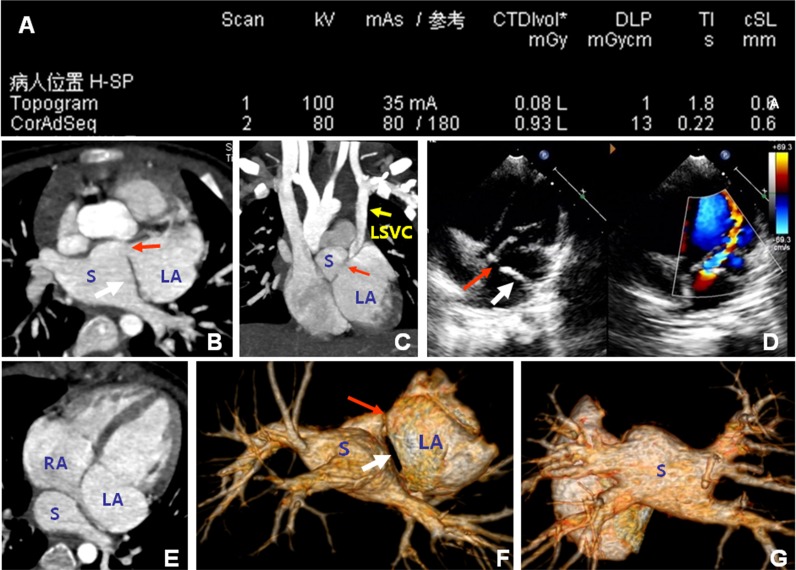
Representative multi-slice spiral computed tomography images from a female patient aged 2 years. A. Scanning parameters. CTDIvol, volume computed tomography dose index; DLP, dose length product (DLP). The effective radiation dose was calculated to be 0.018×2.3×13 = 0.538 mSv. The maximum density in B, C, and E revealed a membrane in the left atrium (white arrow), which separated the left atrium into the left atrium proper (LA) and a split atrium (S). Non-continuous perforated holes existed on the anterior part of the membrane (red arrow). The left superior vena cava is shown (yellow arrow). D. TTE revealed the membrane in the left atrium (white arrow) and communicating holes (red arrows). F and G. VR images show that the membrane separated the atrium into two chambers. The communicating holes were present at the anterior part of the membrane, and that left and right pulmonary veins were led to the split atrium (S). LA, left atrium; S, split atrium; RA, right atrium; LSVC, left superior vena cava; TTE, trans-thoracic echocardiography; VR, volume rendered.
